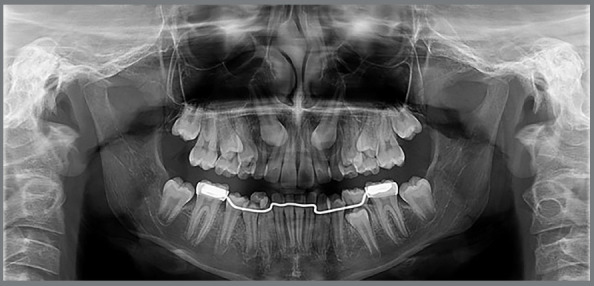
Initial panoramic radiograph with cemented lingual arch.
